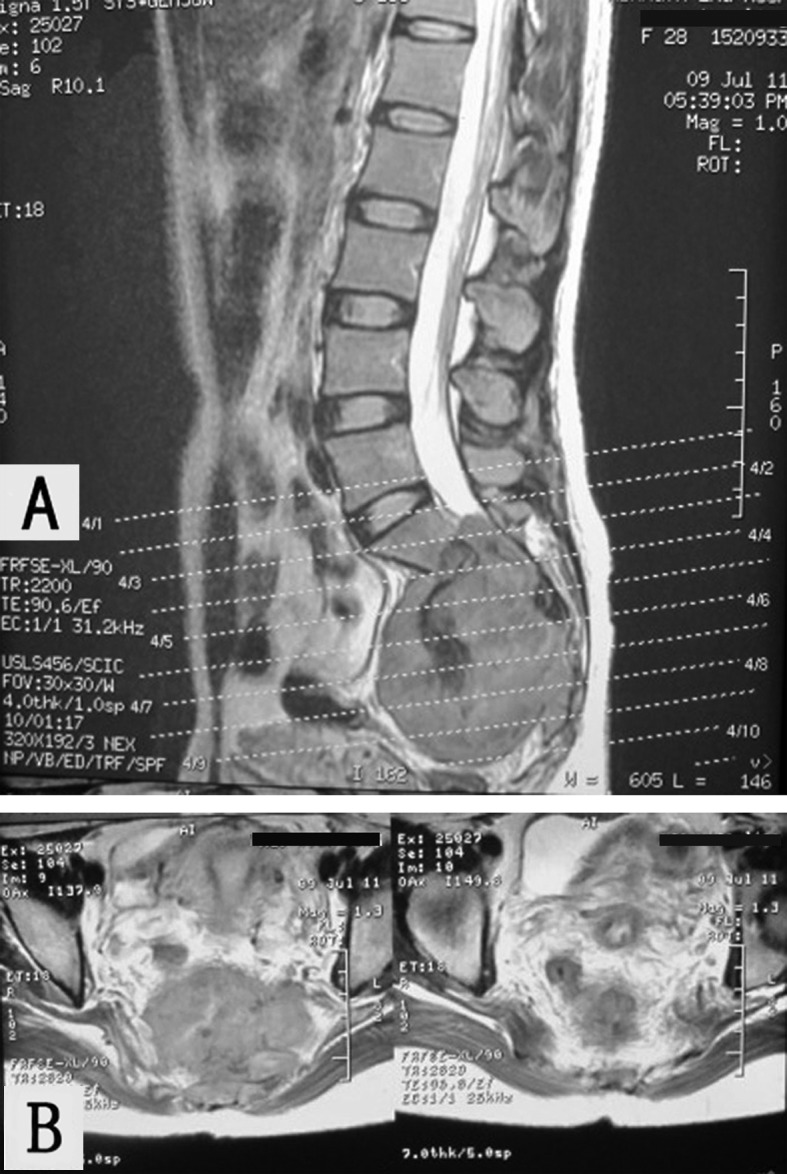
(A and B) Magnetic resonance images (MRI) showing the destruction of the sacrococcygeal bones and a huge soft-tissue mass. On T1- and T2-weighted images, the lesion showed large patches of mixed signal shadows, which followed the plane of the first sacral vertebra. (A) The boundary between the mass and the rectum was unclear. (B) Upon enhanced scanning, the lesions appeared more pronounced.
